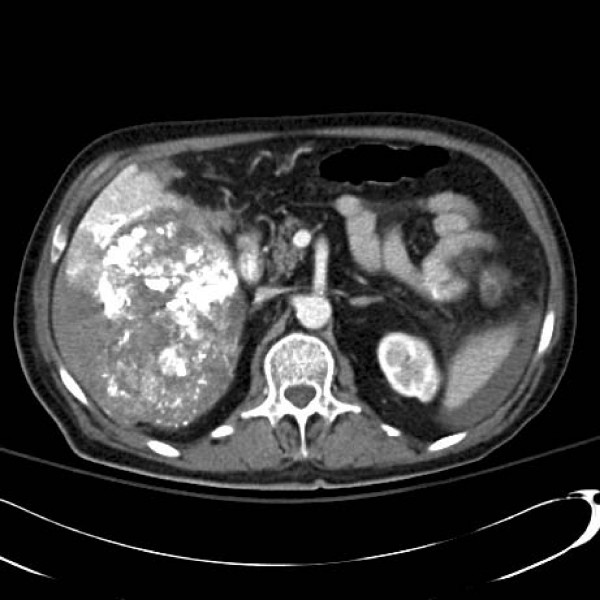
Computed tomographic scan of ruptured hepatocellular carcinoma post-TACE.
